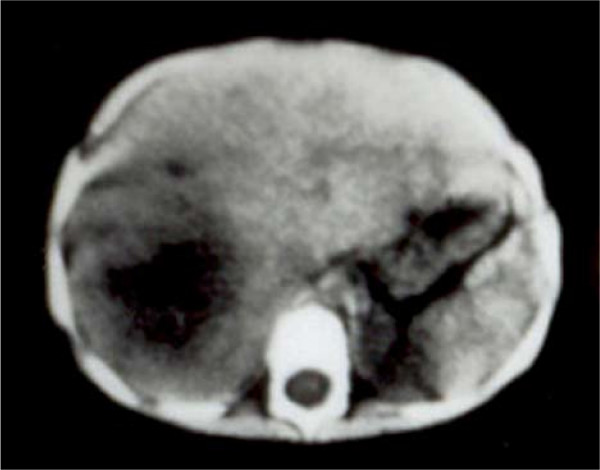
Computed tomography scan of the liver showing the liver abscess.
